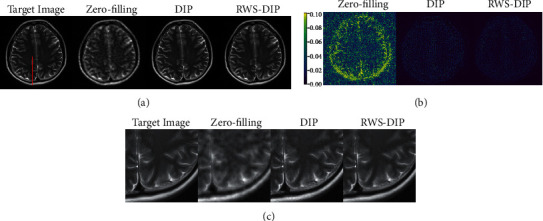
Comparison of reconstructions of the target MR image in Brain C using the variable density undersampled mask with 30% sampling rate: (a) the target image and reconstruction results, (b) the corresponding error images, and (c) the corresponding zoom-in images.
